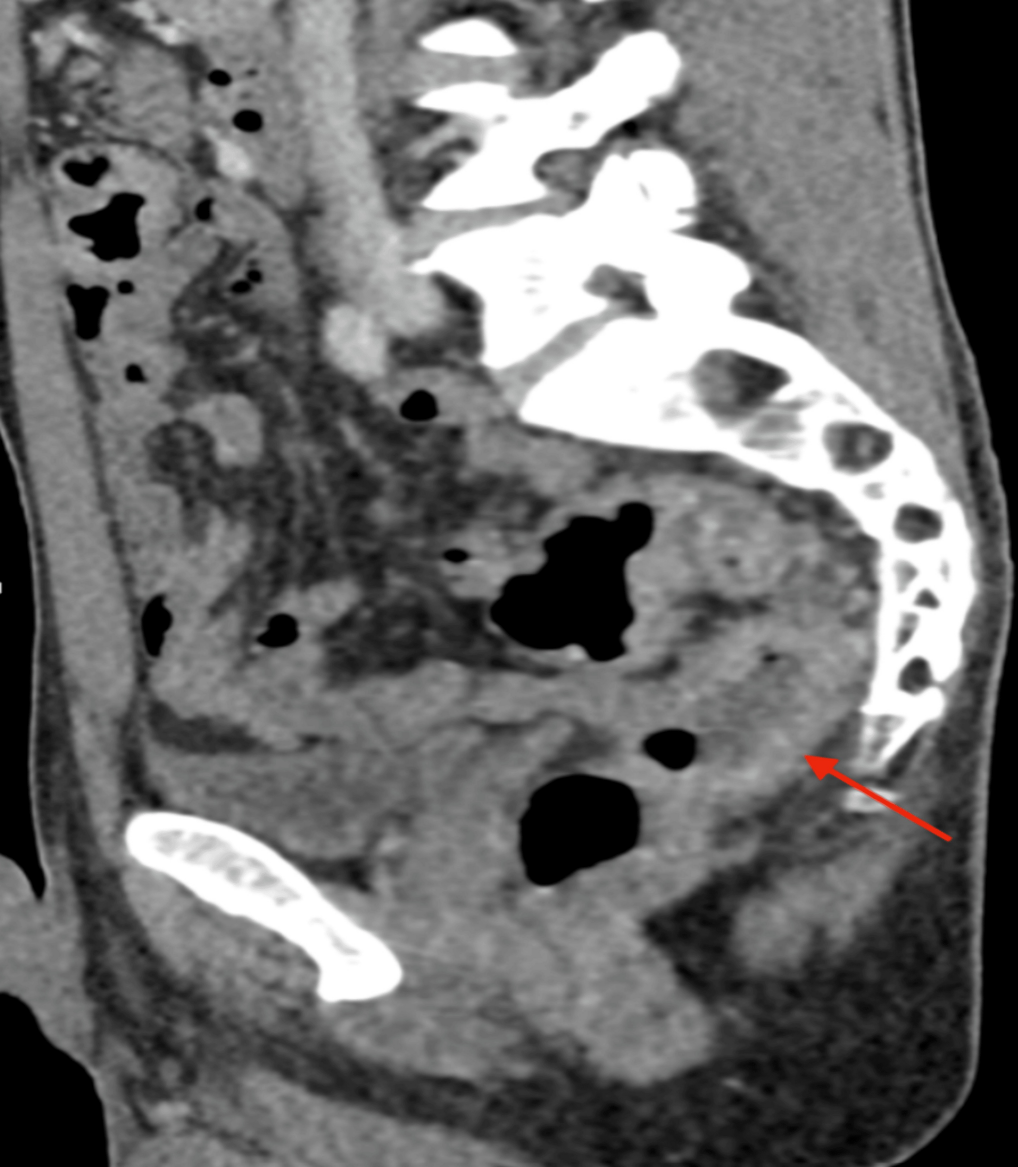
Sagittal CT imaging showing coloproctitis Sagittal reformat image of the abdomen and pelvis with IV contrast demonstrates mild wall thickening and enhancement involving the rectum and sigmoid colon, consistent with colitis.
